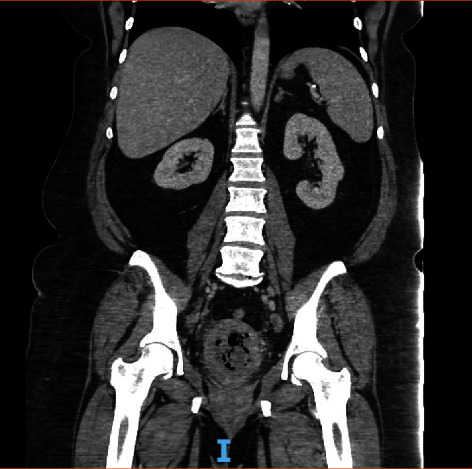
CT scan with contrast at time of presentation to the emergency department. Gas fluid collection was noted to measure up to 13 cm in length, 6 cm in width, and 5 cm in height. Findings concerning for abscess/pyometra.
